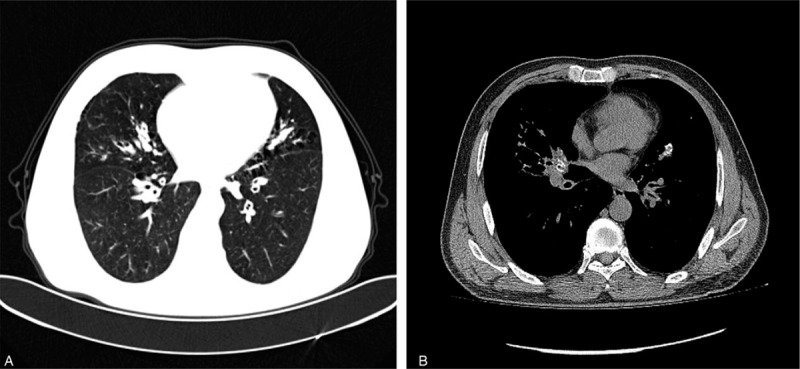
High-resolution chest CT showing right lung middle lobe atelectasis with diffused calcification and a thick-walled cavity in the bilateral bronchi (1A and 1B).
